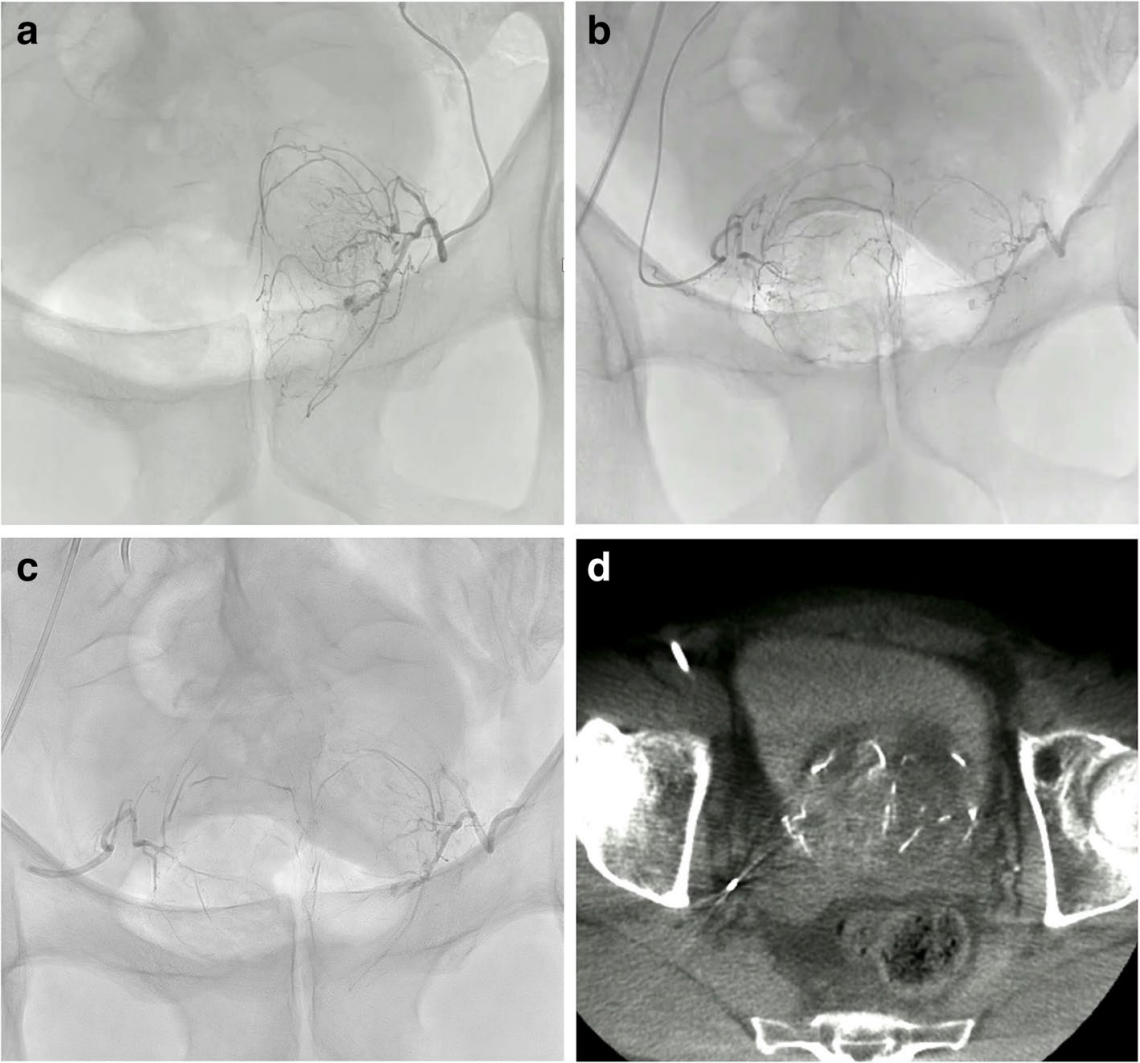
Seventy one-year-old man referred for prostatic artery embolisation (PAE) to treat lower urinary tract symptoms (LUTS) due to benign prostatic hyperplasia. a Selective left prostatic artery angiography with a 2.4-Fr microcatheter before PAE showing left prostatic lobe enlargement with a normal distal vascular bed. b Selective right prostatic artery angiography after PAE of the left prostatic artery with AHCA-MagicGlue®/Lipiodol® in a 1:8 ratio visualising the blood supply to the right prostatic lobe and demonstrating complete occlusion of both the distal and the proximal segments of the left prostatic artery. c Control angiogram after PAE of the right prostatic artery using the same mixture: note the cast in the distal and proximal branches of the right prostatic artery, with complete occlusion. d Axial cone-beam computed tomography with contrast injection after bilateral PAE: Lipiodol® uptake by both prostatic lobes with distal and proximal distribution of the AHCA-MagicGlue®/Lipiodol.® casts. Major improvements in the LUTS were noted 3 months after PAE compared to baseline (International Prostate Symptoms Score (IPSS), 4 vs. 21; IPSS-Quality of Life, 2 vs. 6; prostate volume, 59 vs. 91 mL)
